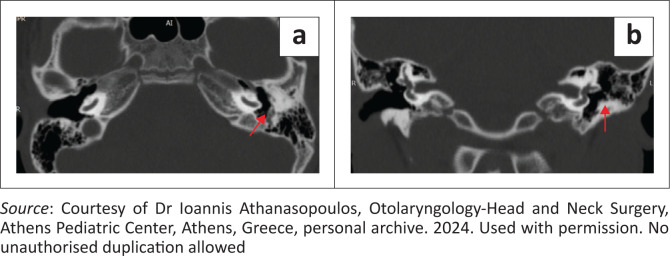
Left external auditory canal (EAC) atresia in a 7-year-old male patient. (a) Axial CT image demonstrates complete bony atresia of the left EAC with an absent canal lumen and a small, dysplastic middle ear cavity (red arrow). (b) Coronal CT image further shows the atretic EAC (red arrow).
